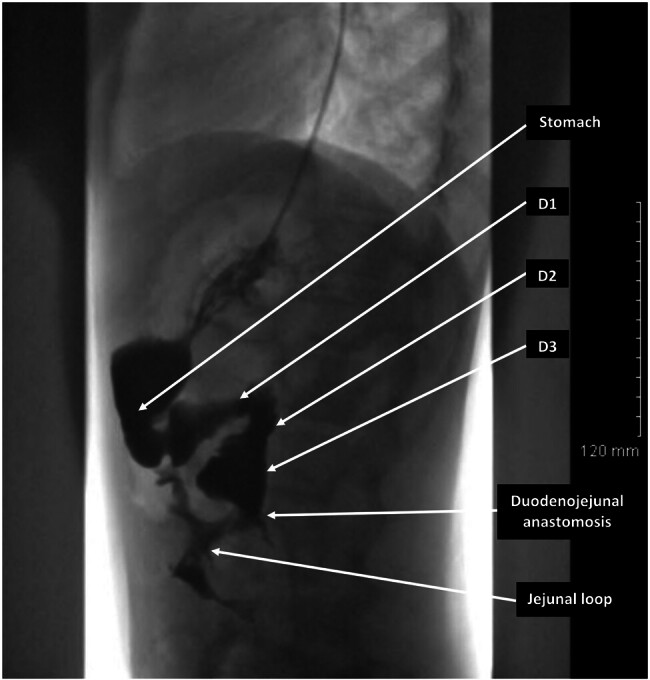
Lateral view of an upper gastrointestinal tract radiography performed 10 days after surgery, showing normal gastric and duodenal evacuation within a short time, with the persistence of slight distension in the first and second portions of the duodenum upstream of the anastomosis. D1, first portion of the duodenum; D2, second portion of the duodenum; D3, third portion of the duodenum.
